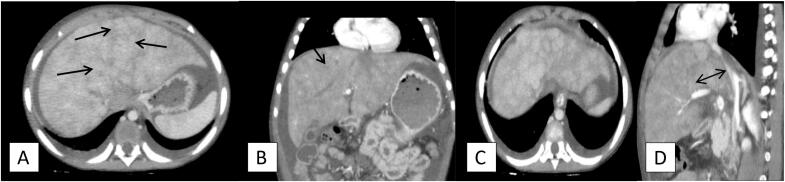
CT findings: (A, B) Axial and coronal CT post contrast image shows non-opacified hepatic veins (black arrows in the image A and B) with mild splenomegaly and moderate ascites C) axial post contrast image: Mildly enlarged liver with heterogeneous hypo attenuation and patchy enhancement (nutmeg liver) with minimal bilateral pleural effusion. d) Sagittal post contrast images: focal intrahepatic IVC narrowing (double headed arrow on D).
